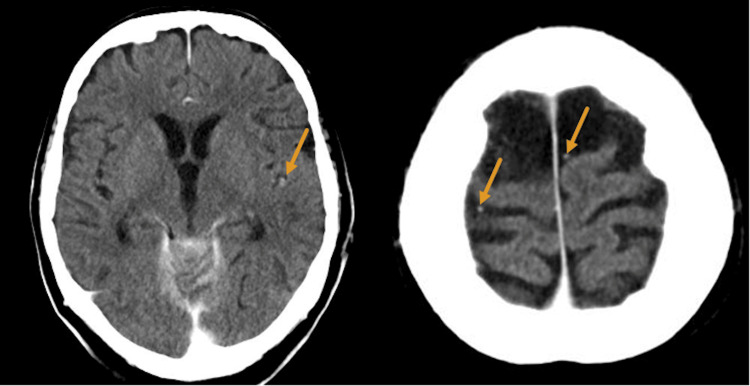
Brain computed tomography revealing suspected metastasis (arrows).
